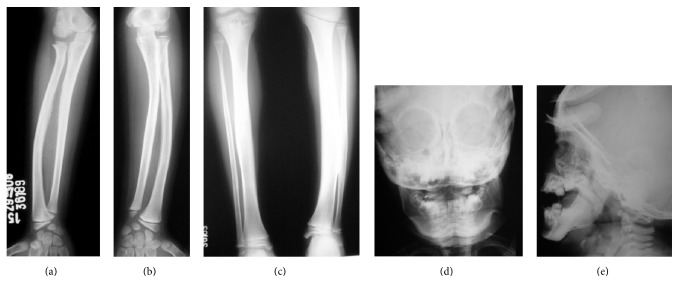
Radiographs showing spontaneous resolution. ((a) and (b)) Radiograph findings of right and left forearms were normal in follow-up examination at eight years of age. (c) The roentgenographic findings of tibias and left fibula were normal in follow-up examination at eight years of age. ((d) and (e)) Swelling of mandible had diminished and disappeared completely at 1 + 2 years of age (frontal and lateral projection).
